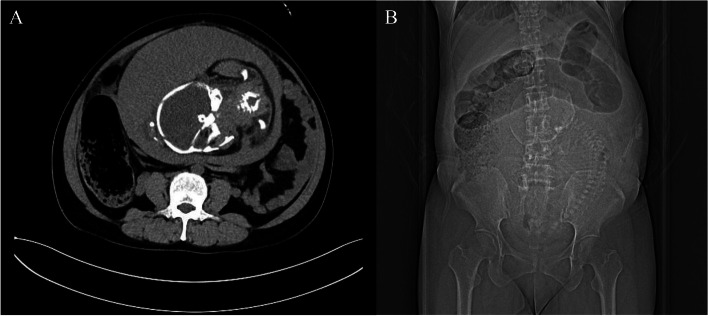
Axial (A) and coronal (B) views of computed tomography (CT) of the abdomen and pelvis. CT demonstrated gross distension of ascending colon and transverse colon
